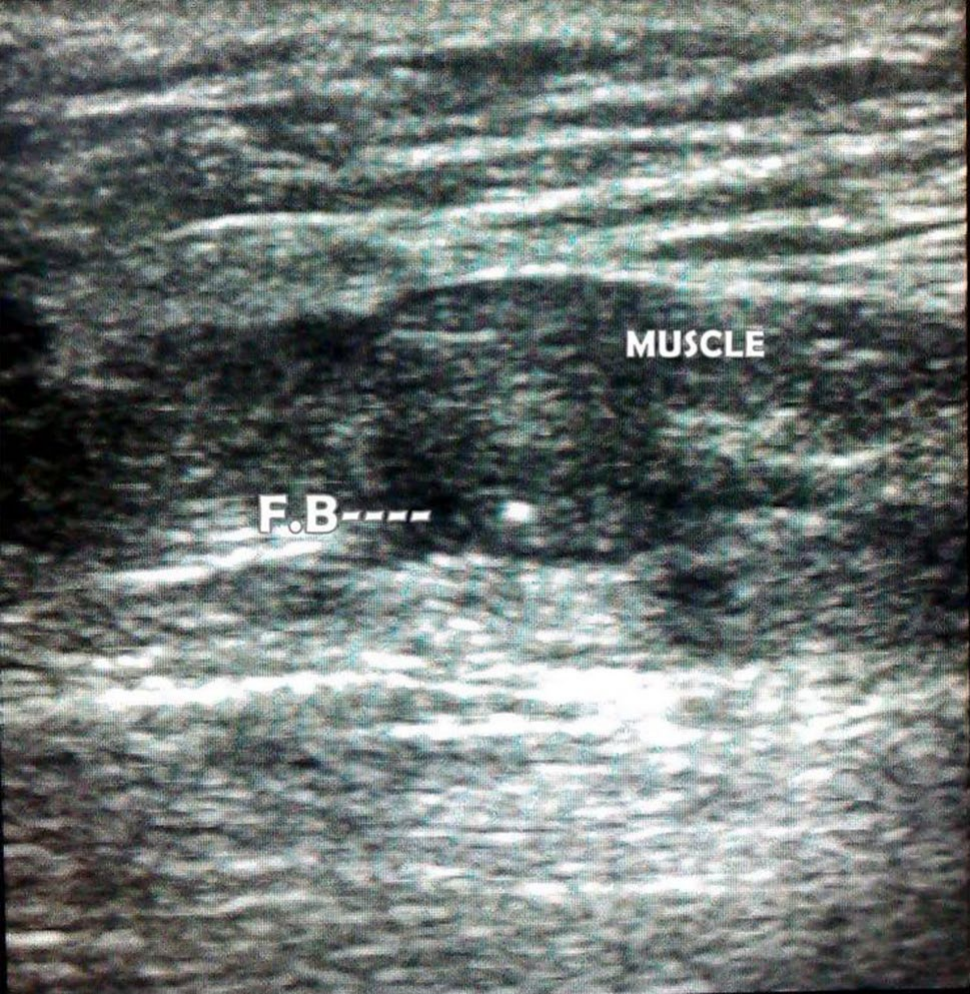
Ultrasound of calf showing foreign body within the muscle
